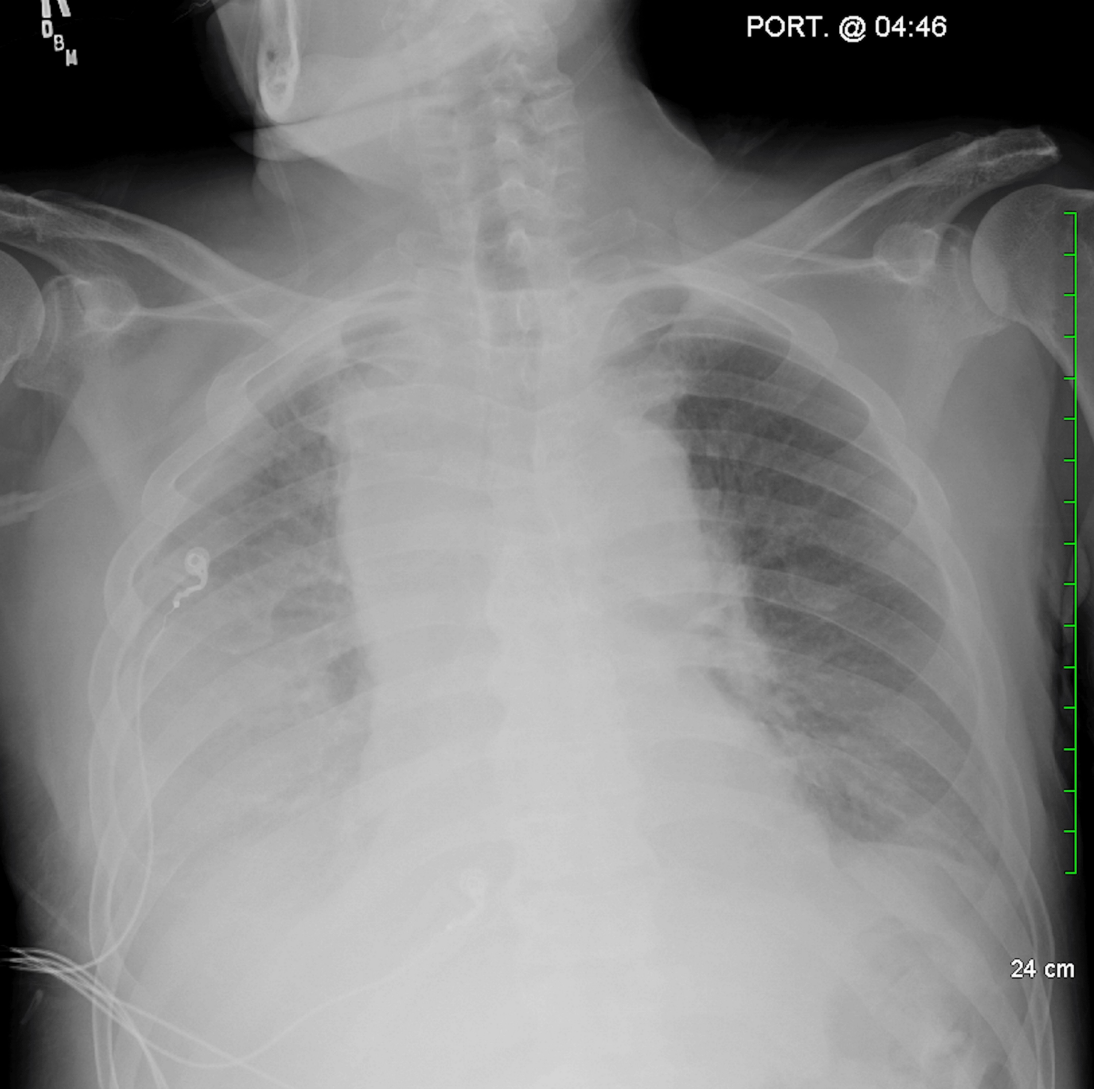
The chest X-ray shows a right pleural effusion, which appears to have increased in size compared to the previous CT scan. There is also mediastinal widening with soft tissue density, consistent with the patient’s known history of lymphadenopathy. CT: computed tomography.
